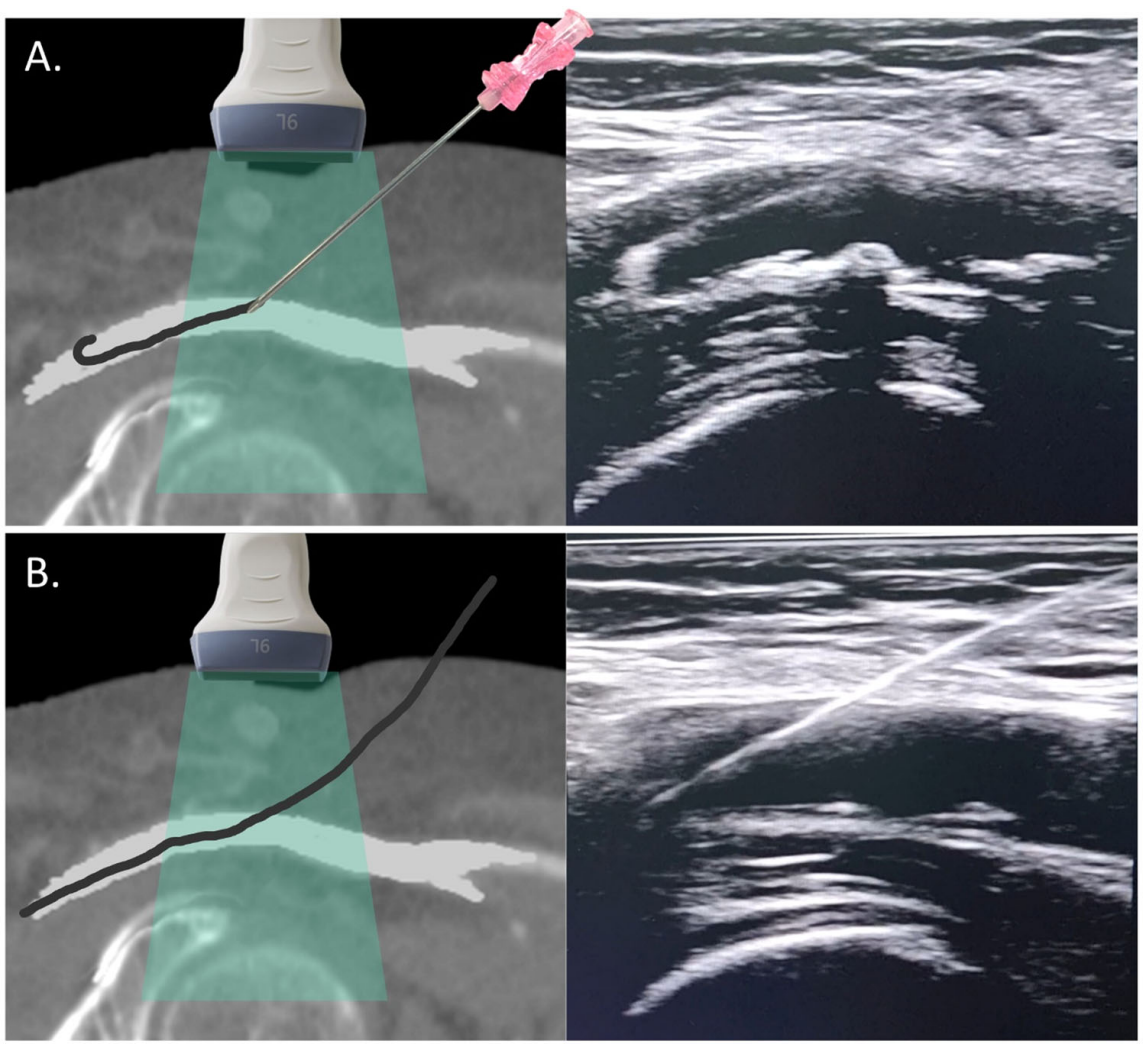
Confirmation of guidewire position in the true lumen and entry point (Step 6). (A) After successfully puncturing the CFA anterior wall, the operator can visualize in real‐time the guidewire advancement of the guidewire into the true lumen of the vessel under ultrasound guidance. (B) Following needle removal, the operator uses ultrasound to reassess the CFA at the puncture site, ensuring proper location and vessel integrity before sheath insertion. [Color figure can be viewed at wileyonlinelibrary.com]
